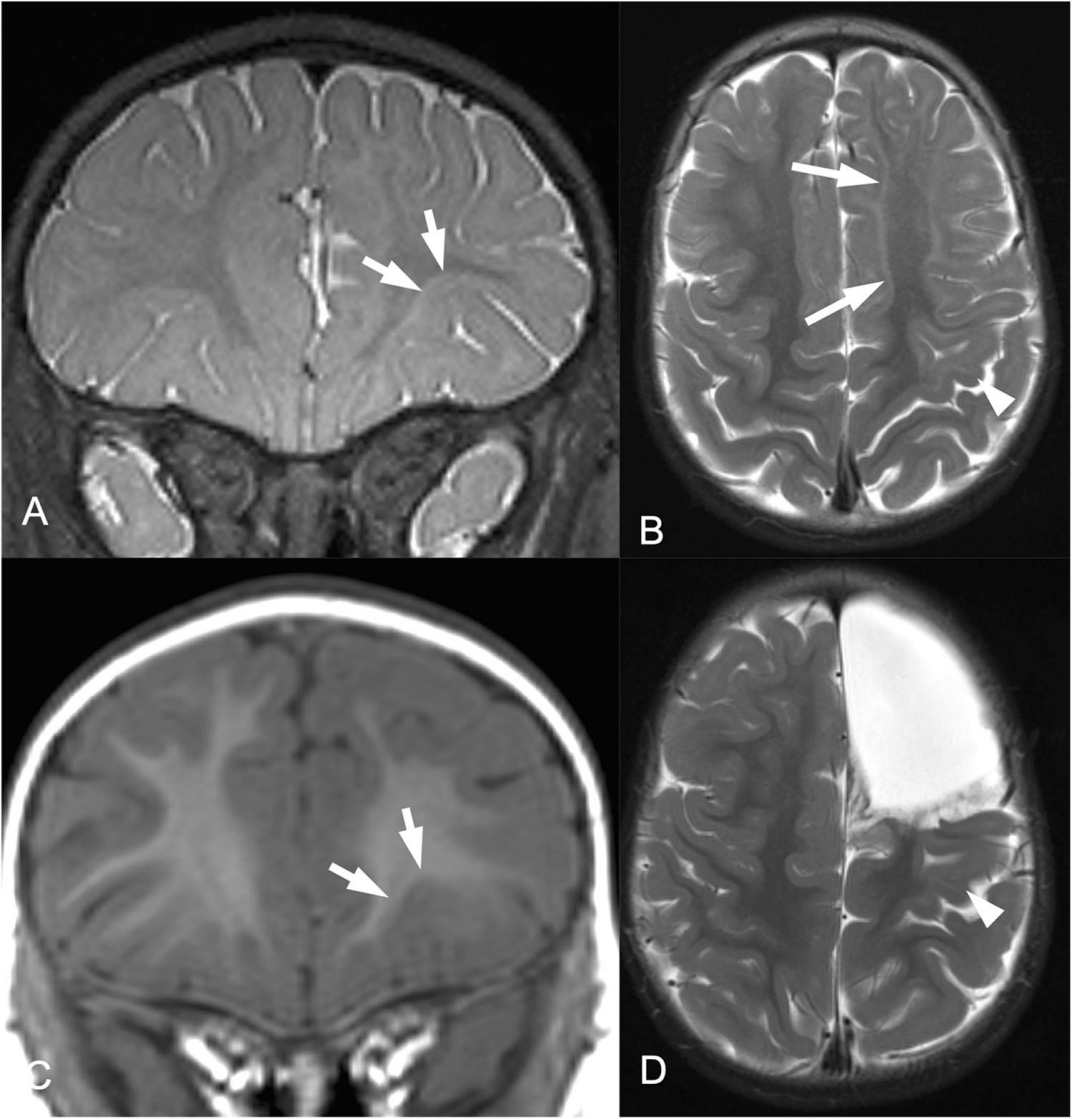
Mild malformation of cortical development with oligodendroglial hyperplasia (MOGHE) in the left frontal lobe in a 3-year-old girl. A–C 3 Tesla coronal 3D T2 SPACE (A), axial T2 TSE (B), coronal T1 MPRAGE (C), and axial T2 TSE (D) images. T2-weighted images show a juxtacortical hyperintense signal band (A–B, arrows), extending into the left precentral gyrus (B, arrowhead). On the MPRAGE image, the T2-hyperintense band looks like a subtle blurring of the gray-white matter junction (C arrows). D Axial T2w image after subtotal left frontal lobe resection. Despite residual lesion in the left precentral gyrus, the patient was seizure-free (Engel IA) after 3 months
